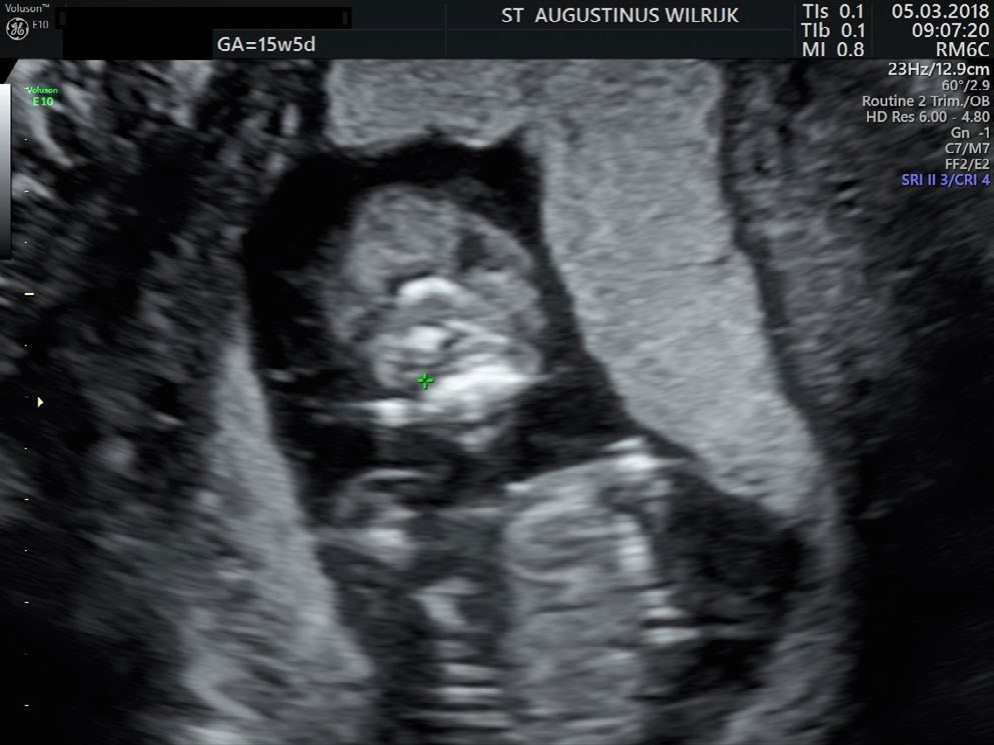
Ultrasound at 15 wks and 5 d shows the impression of a unilateral cleft lip
